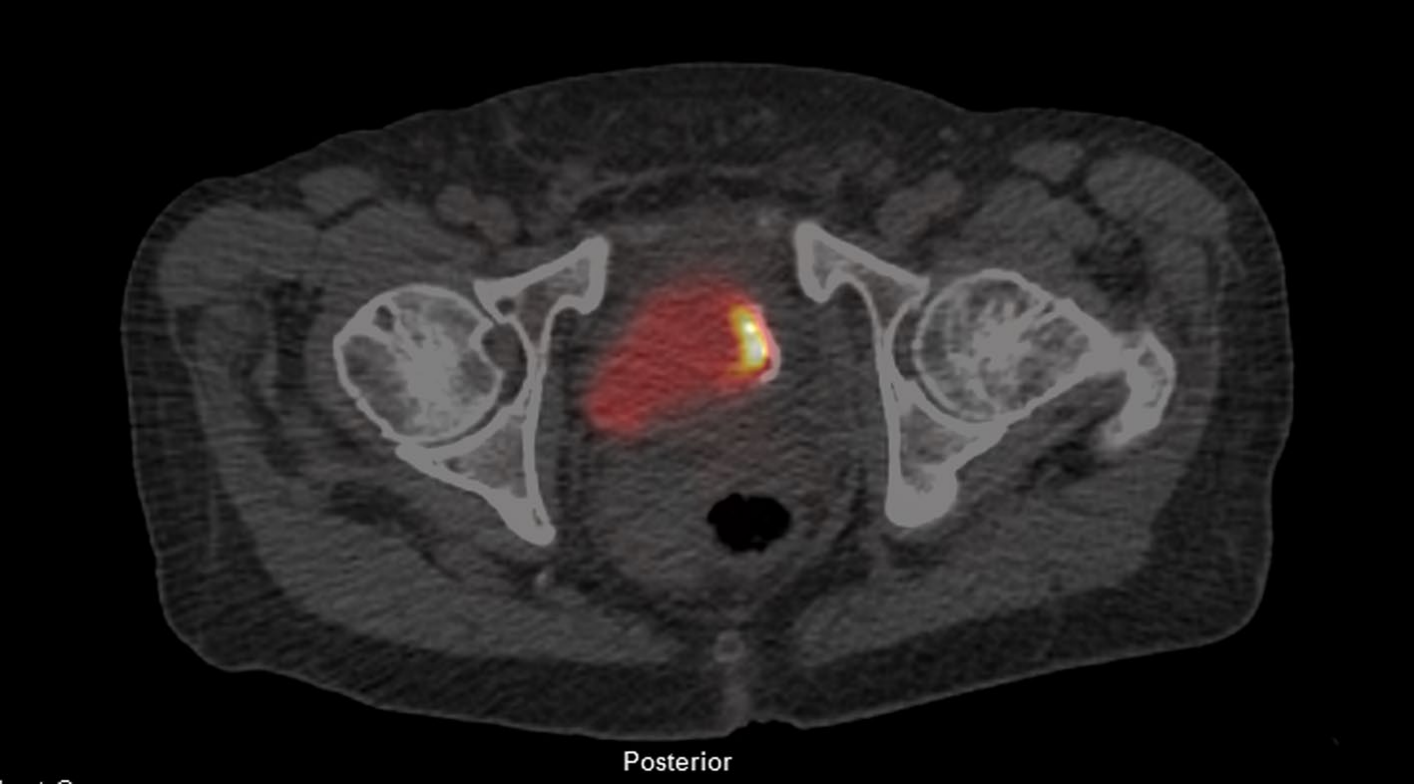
Fused transaxial images of FDG PET/CT with intense FDG uptake in the left lateral urinary bladder wall (SUV_max_ = 67.9) and calcification, at the prior resected and treated DLBCL
